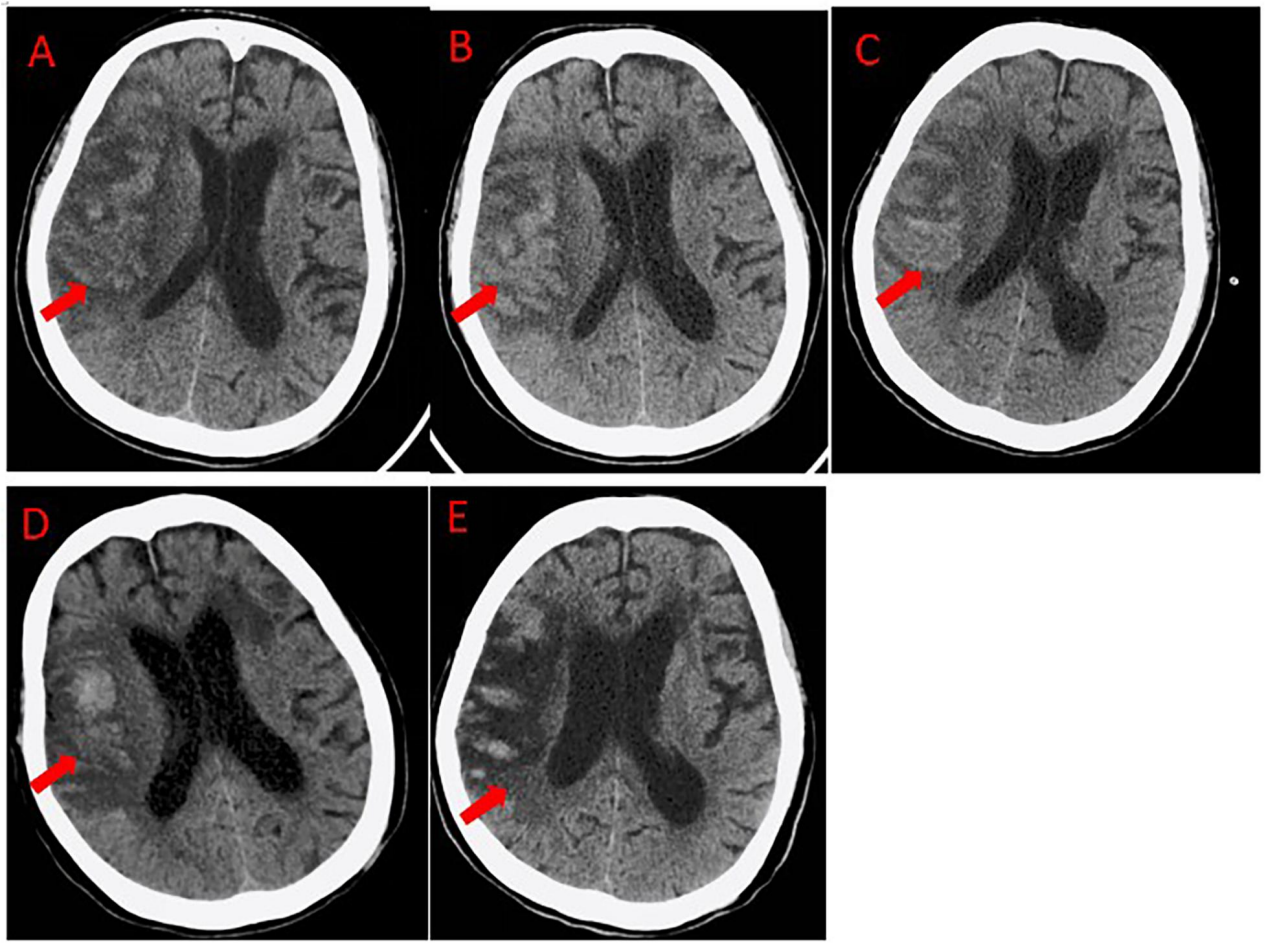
Non-contrast head CT images. (A) Day 4 after cerebral thrombectomy: initial development of patchy high-density areas (arrows), indicative of early hemorrhagic transformation. (B) Day 6 after cerebral thrombectomy: progressive expansion and coalescence of the hemorrhage (arrows). (C) Day 12 after cerebral thrombectomy: slight reduction in the size and density of the hemorrhage (arrows). (D) Day 17 after cerebral thrombectomy (pre-pulmonary intervention): re-expansion of the hemorrhage (arrows). (E) Day 7 after pulmonary thrombectomy: stability of the intracranial hemorrhage, confirming no procedure-related exacerbation (arrows).
